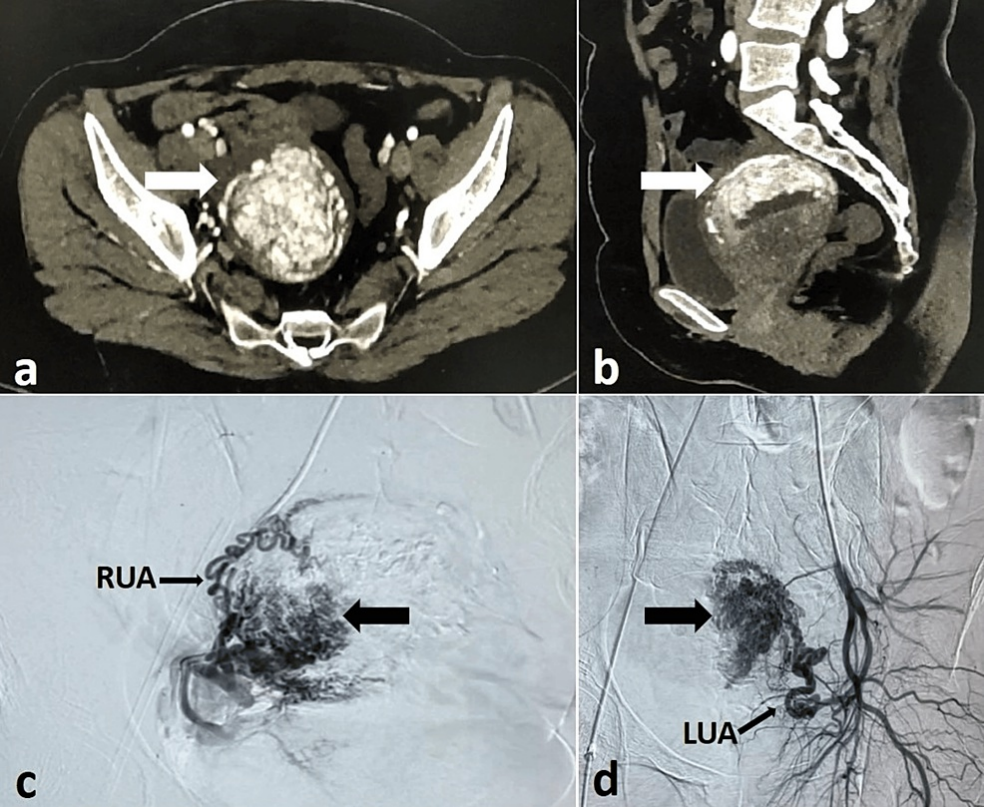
CT and pre-embolization DSA images of the patient with AVM CECT arterial phase axial (a) and sagittal (b) images showing an AVM (white arrows) involving the anterior myometrium. (c, d) Pre-embolization DSA images showing the low flow AVM (thick black arrows). AVM, arteriovenous malformation; CECT, contrast-enhanced CT; DSA, digital subtraction angiography; LUA, left uterine artery; RUA, right uterine artery
